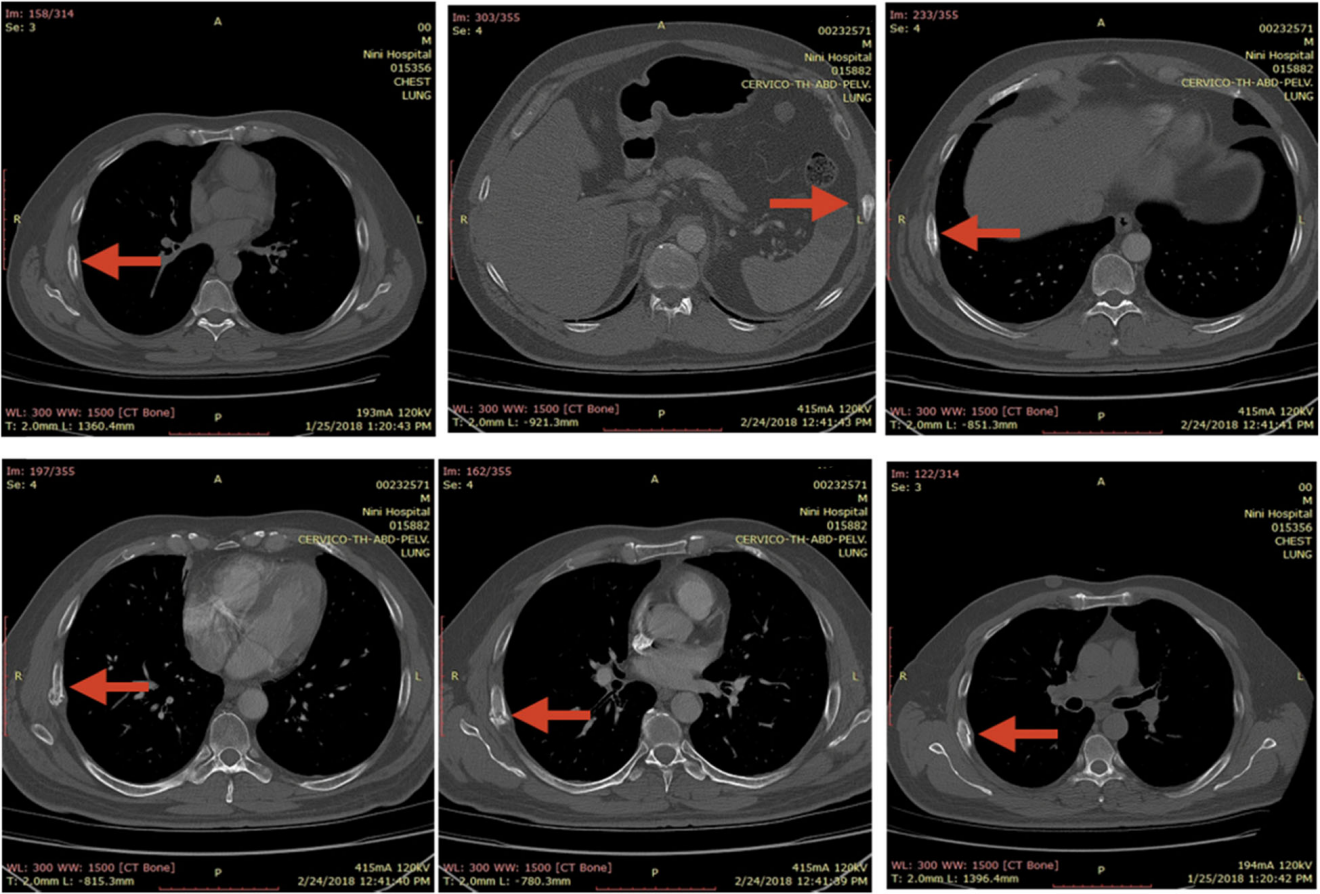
Chest computed tomographic scan shows fractures of fifth, sixth, seventh, and ninth left ribs and the anterior bow of the fifth right rib *(red arrow)*. Dr. Bassel El Osta (25 Jan 2018), Tripoli: Nini Hospital
